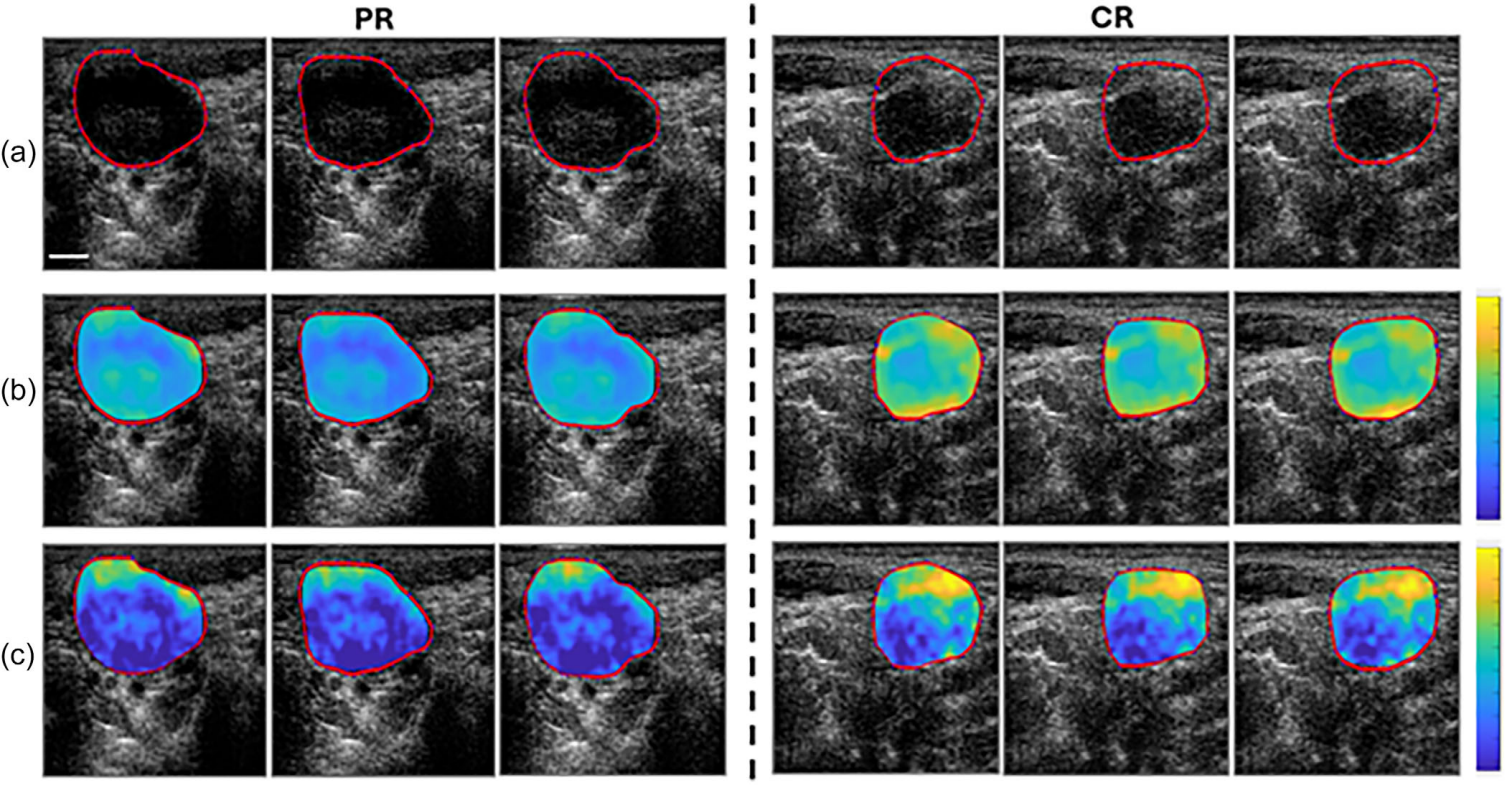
Representative QUS parametric maps of three CR patients (On the left) and three PR patients (On the right). (a) Presents the B‐mode ultrasounds with ROIs. (b) Mid‐Band fit parametric maps (range from −10 to 40 dB). (c) Spectral intercept parametric maps (range from 10 to 50 dB). Scale bar is 5 mm.
